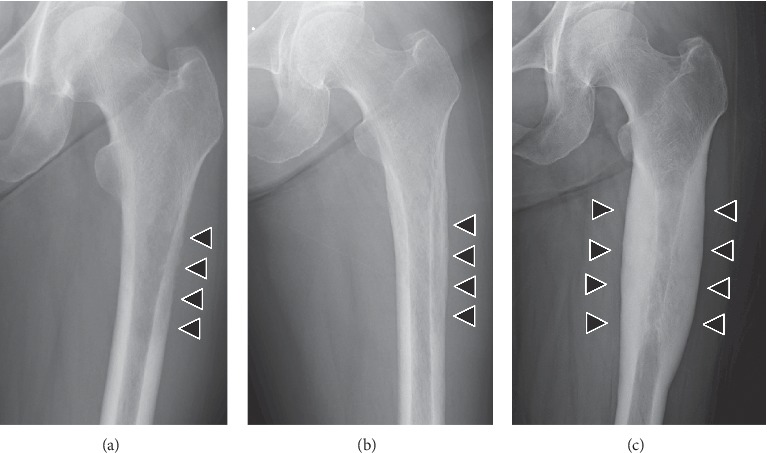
X-ray findings of the left femur at different timepoints showing the radiologic change of a purely osteolytic lesion to an osteosclerotic lesion over time (arrowhead). (a) At first visit, (b) at 3 months after the first visit, and (c) at 15 months after the first visit.
